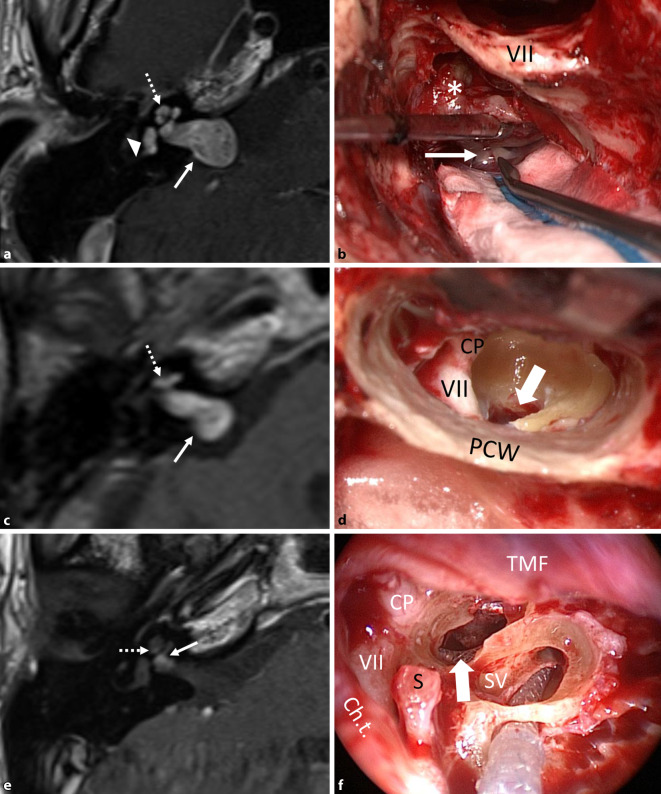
a,b Patient 1 in Table 2. a Magnetic resonance imaging (MRI, axial, T1-w + gadolinium, Gd) showing the transotic tumor with typical gadolinium enhancement in the entire inner ear, IAC and CPA (→), (middle ear extension not shown). b Intraoperative picture showing the facial nerve canal in the mastoid (VII) after lateral petrosectomy and tumor (*asterisk*) in the IAC + CPA during separation from the facial nerve (→). c,d Patient 2 in Table 2. c MRI (axial, T1-w + Gd) showing the tumor in the cochlea and in the IAC + CPA (→). d Intraoperative view of the fundus of the IAC (→) after combined translabyrinthine and transmeatal-transotic tumor resection. e,f Patient 3 in Table 2. e MRI (axial, T1-w + Gd) showing a transmodiolar ILS with tumor in the second turn of the cochlea and extension into the fundus of the IAC (→). f Intraoperative view after tumor resection from the cochlea with the implant electrode array at the lateral cochlear wall (→). The basal turn was free of tumor. *VII* facial nerve, *Ch.t.* chorda tympani, *CP* cochleariform process; *CPA* cerebellopontine angle, *Gd* gadolinium, *IAC* internal auditory canal, *PCW* posterior canal wall, *TMF* tympanomeatal flap, *S* stapes head, *SV* scala vestibuli, *w* weighted. *Dotted arrow *tumor in cochlea, *triangle* tumor in vestibule
